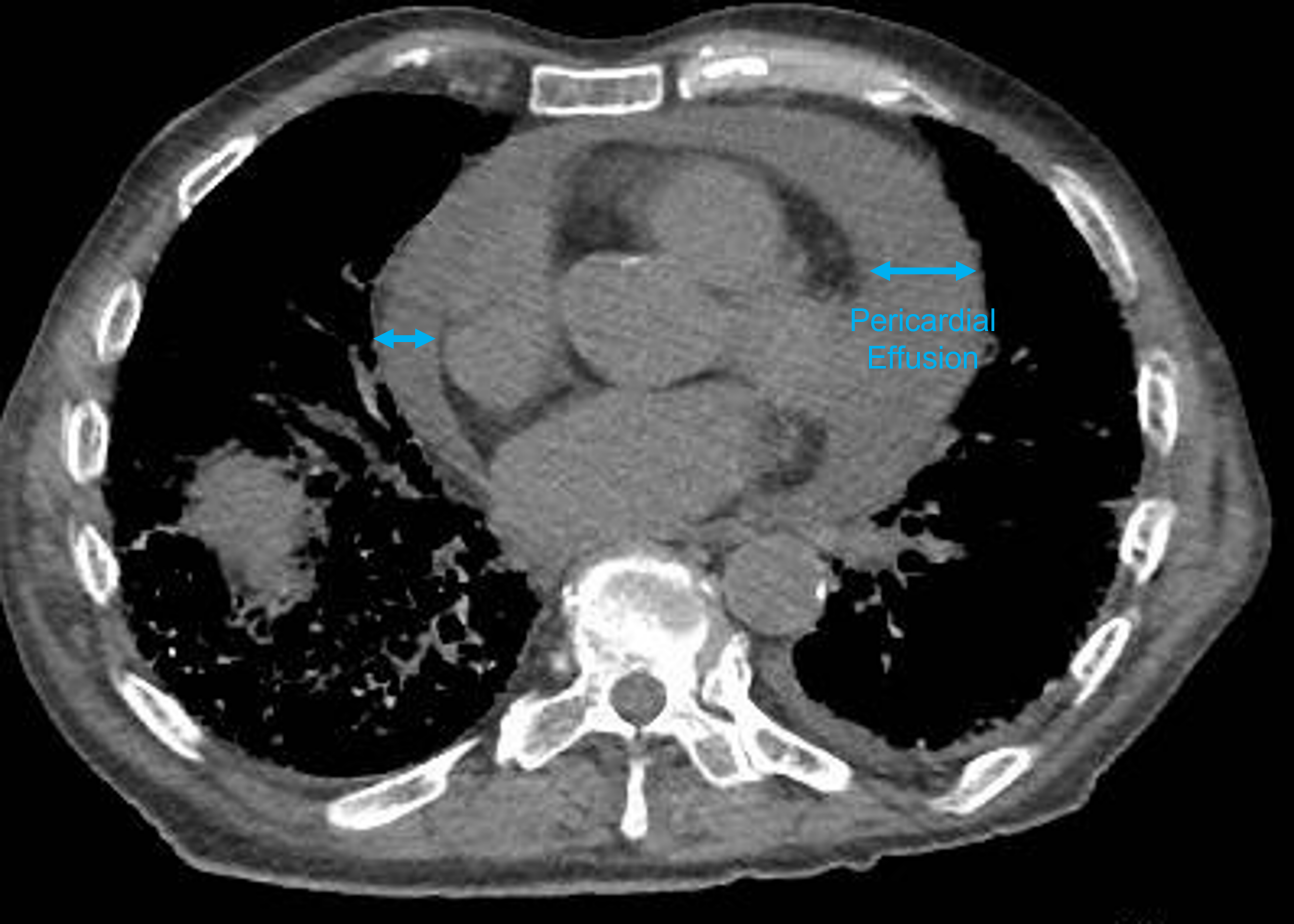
Axial view of computed tomography chest, demonstrating pericardial effusion.
